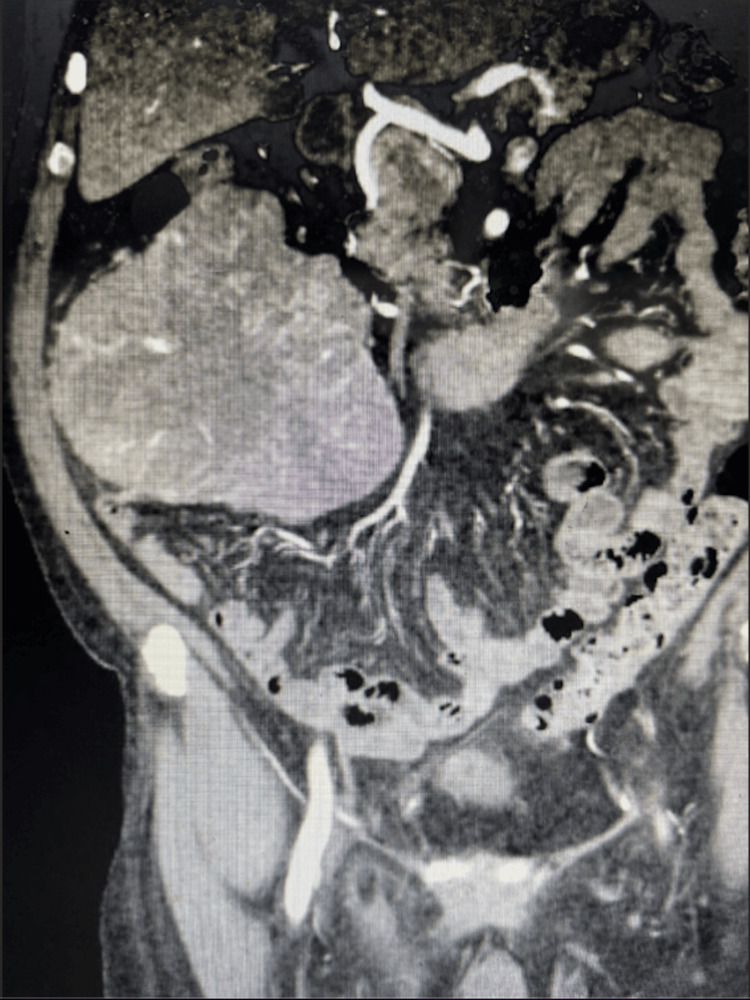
Contrast-enhanced CT scan of the abdomen and pelvis (coronal view) demonstrating a large (13.1 × 9.1 × 14.1 cm) heterogeneously enhancing mass in the right lower quadrant. The lesion exhibits irregular borders, internal heterogeneity, and areas of necrosis, with apparent origin from the mesentery adjacent to the ascending colon. These radiologic features raised concern for a primary mesenteric neoplasm, including sarcoma.
